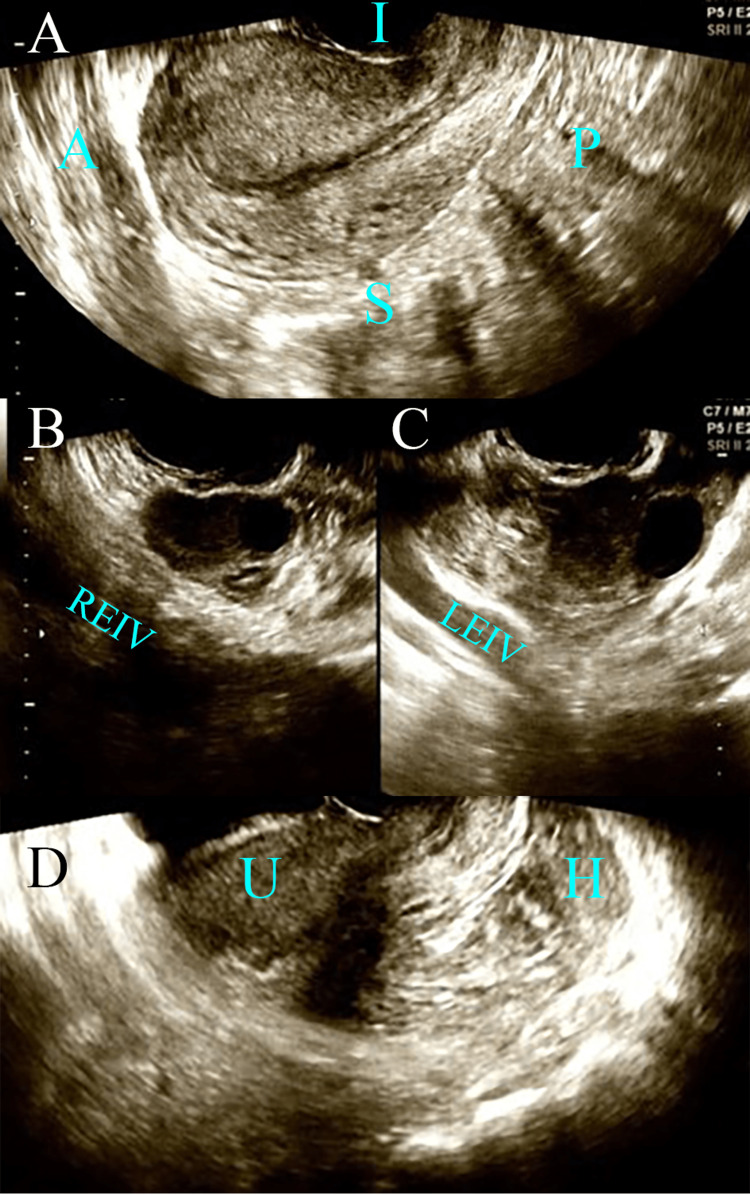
Preoperative images. (A) Transvaginal ultrasound shows thin endometrial lining, indicating no evidence of an intrauterine pregnancy. (B) Normal findings in the right ovary. (C) An inhomogeneous extra-ovarian mass was seen in the left ovary. (D) A hemoperitoneum (free fluid) newly appeared in her cul-de-sac. A, anterior; P, posterior; I, inferior; S, superior; REIV, right external iliac vein; LEIV, left external iliac vein; U, uterus; H, hemoperitoneum.
